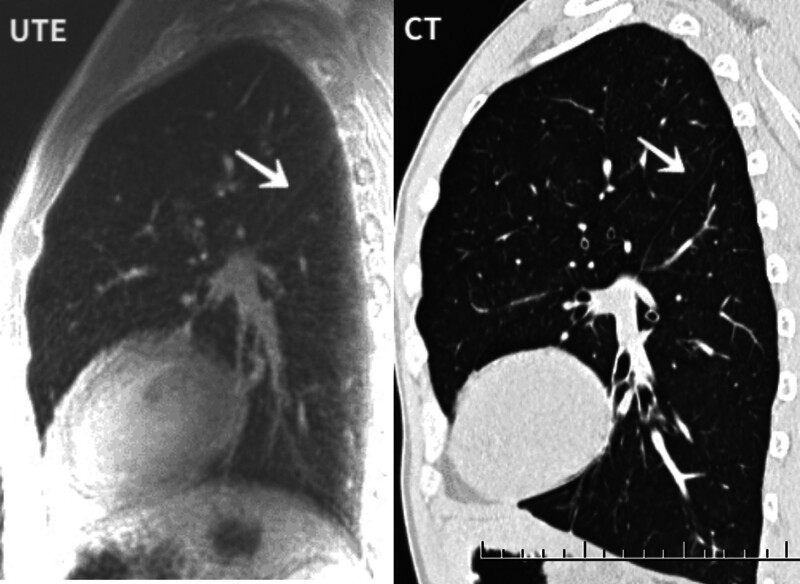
Sagittal view. CT and UTE images were of good overall quality, and the interlobar fissure was clearly visible. UTE = ultrashort echo time.
